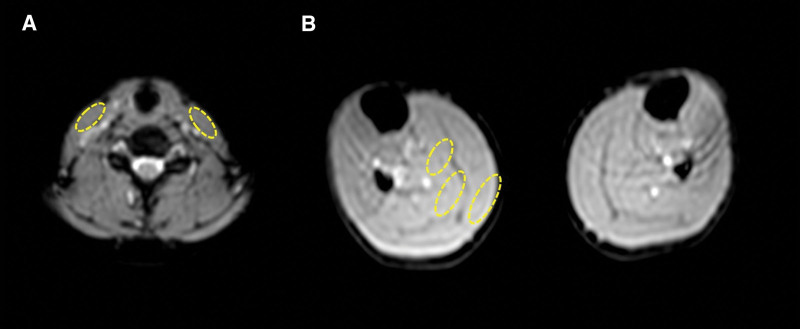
Regions of interest (ROI) in the MR images. (A) The ROI was set in the centre of sternocleidomastoid muscle in the neck area. (B) The ROI was set in three layers, superficial, middle and deep areas of gastrocnemius muscle in the lower leg.
